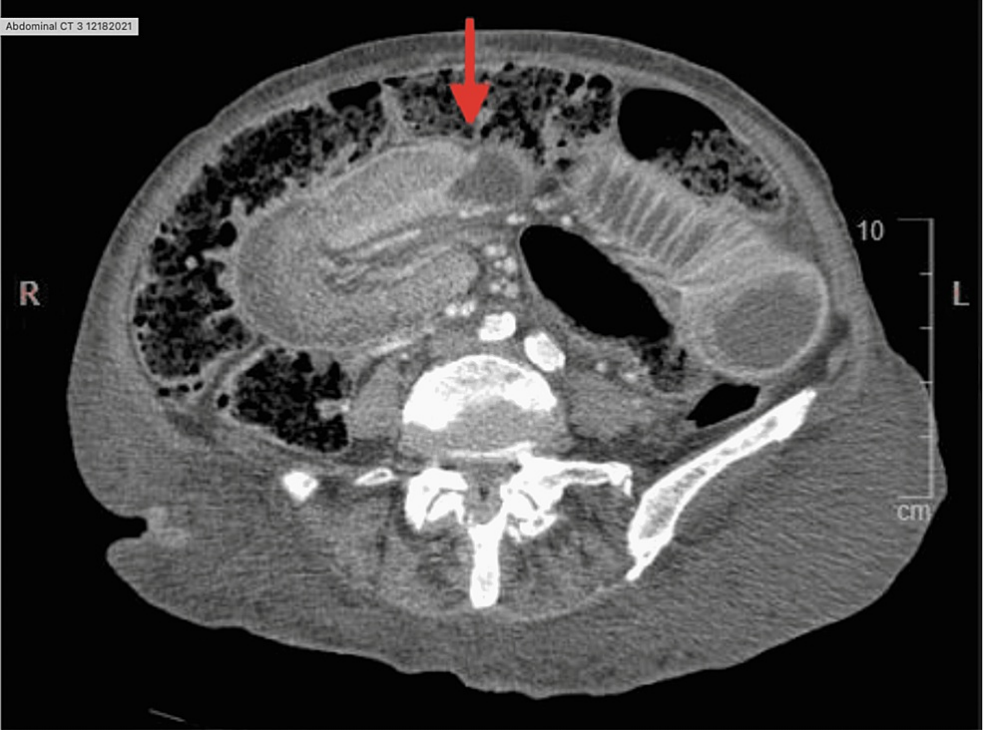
CT with intravenous contrast of the abdomen showing the transition point Red arrow: transition point
